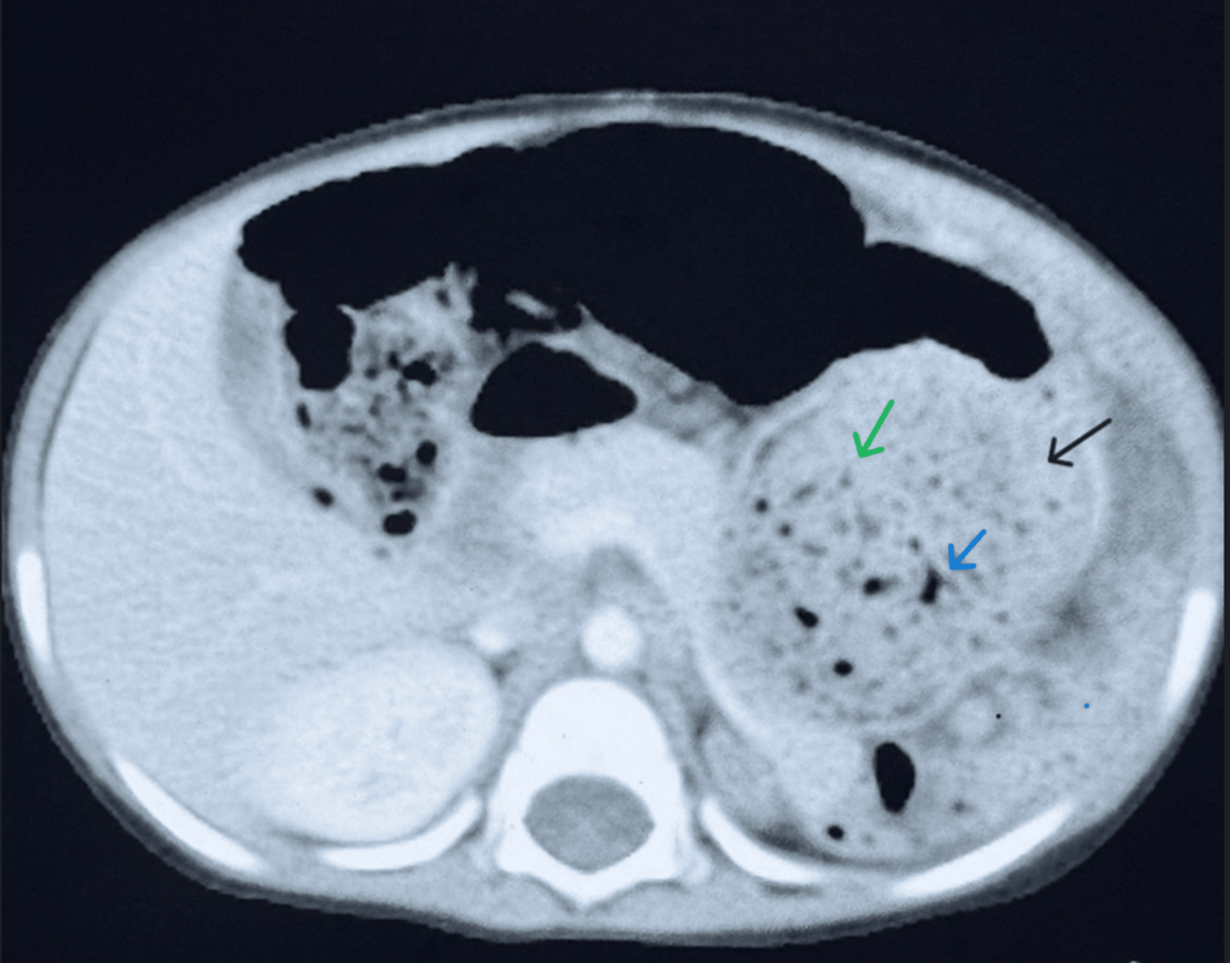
Axial contrast-enhanced computer tomography (CECT) of the abdomen Axial CT image showing a well-defined multiloculated cystic mass (black arrow) with enhancing septa (blue arrow) and solid component (green arrow) in the left upper abdomen, laterally and posteriorly abutting the spleen, anterolaterally abutting the abdominal wall, and medially displacing adjacent bowel loops, pancreas and stomach.
